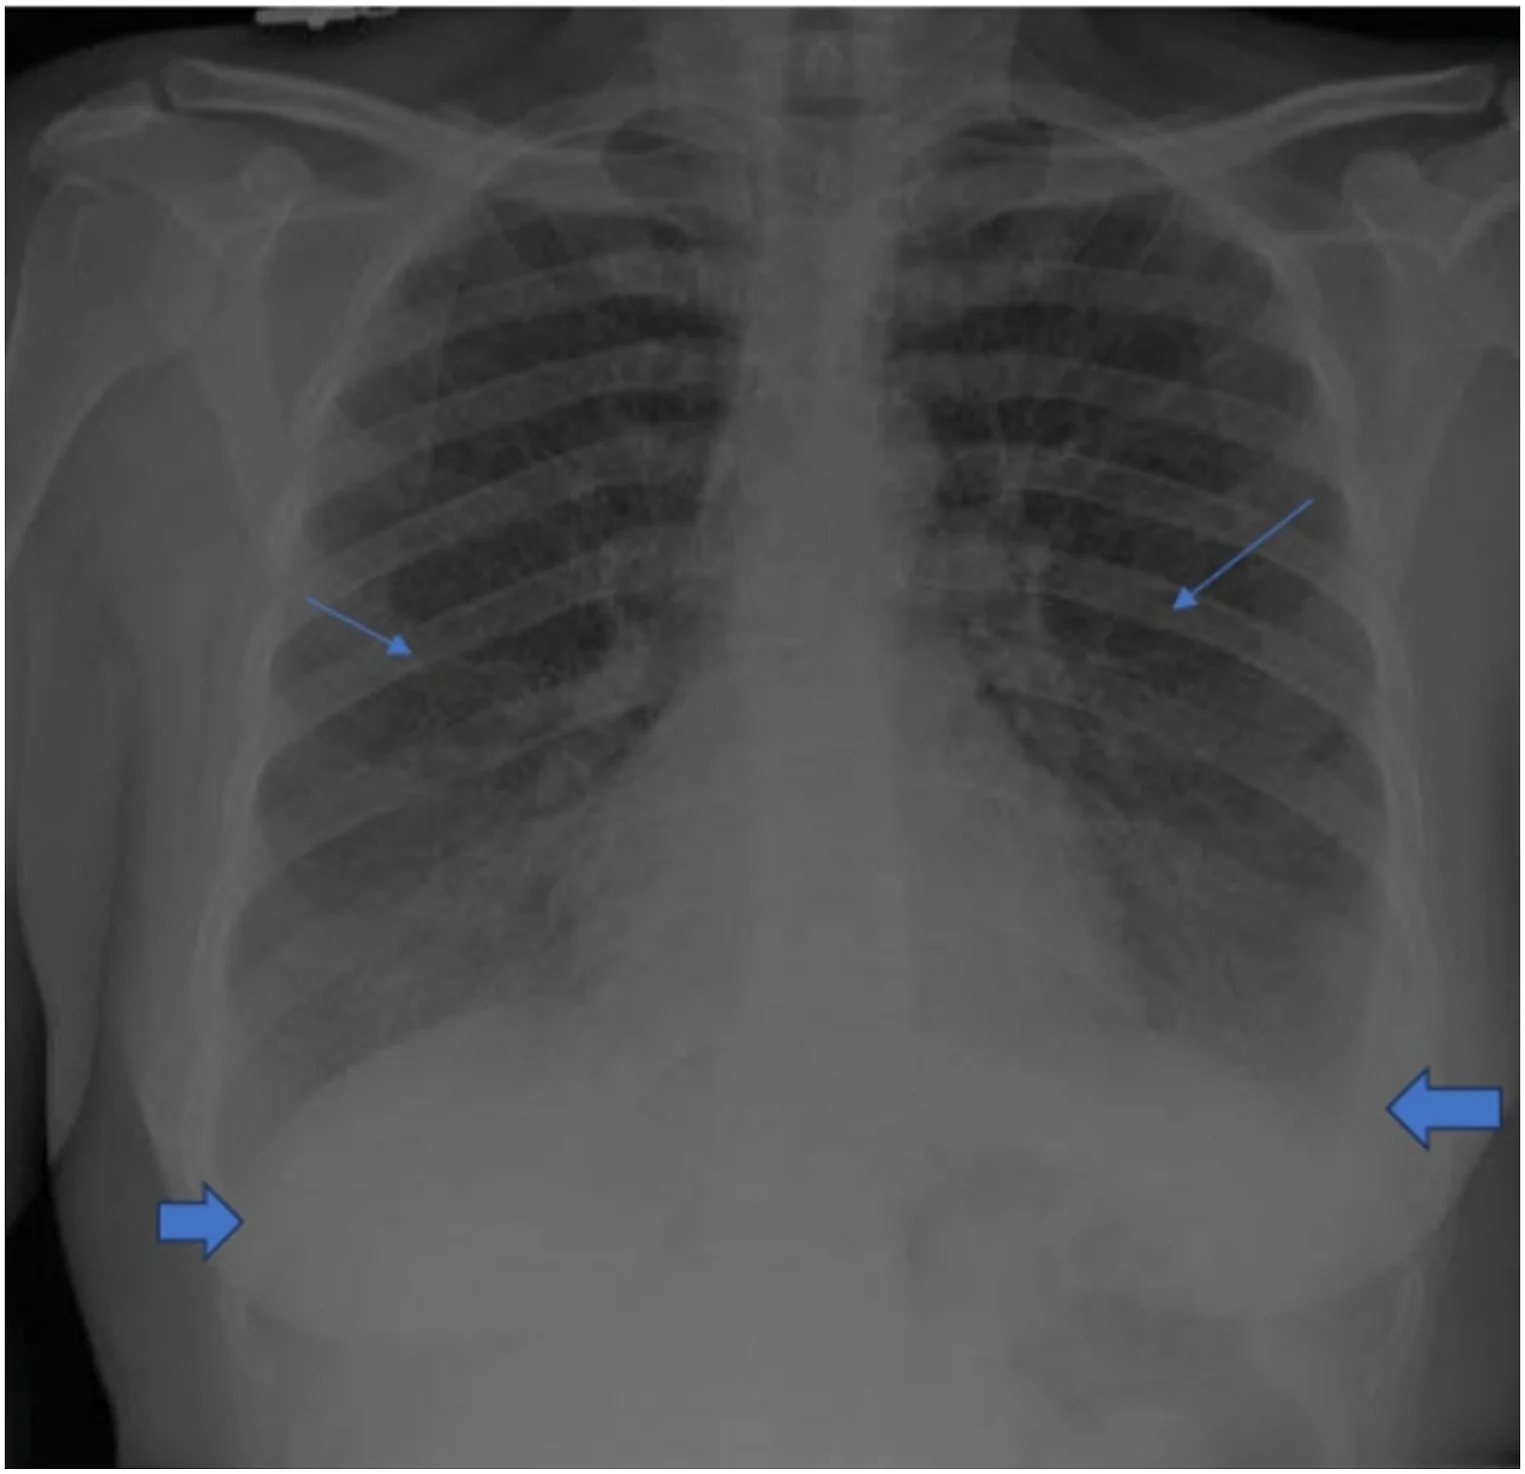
AP chest radiography shows bilateral diffuse reticular pattern (arrows) and bilateral blunted costophrenic angle (block arrows).
